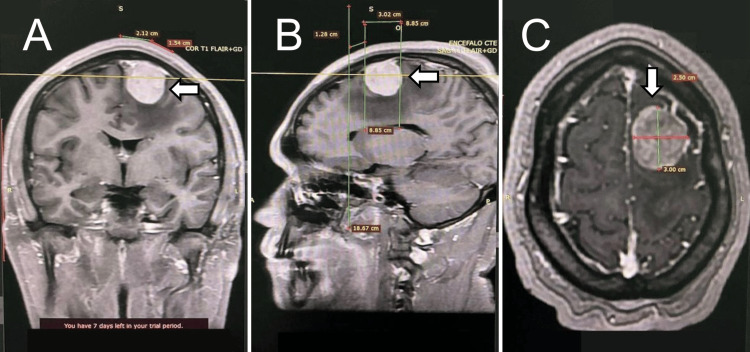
Preoperative contrast-enhanced MRI of a left frontal convexity mass (A) Coronal, (B) sagittal, and (C) axial contrast-enhanced MRI demonstrating an iso- to hyperintense, homogeneous, well-circumscribed, round lesion located at the left frontal convexity, with homogeneous contrast enhancement, surrounding perilesional edema, and a dural tail sign. MRI: magnetic resonance imaging
